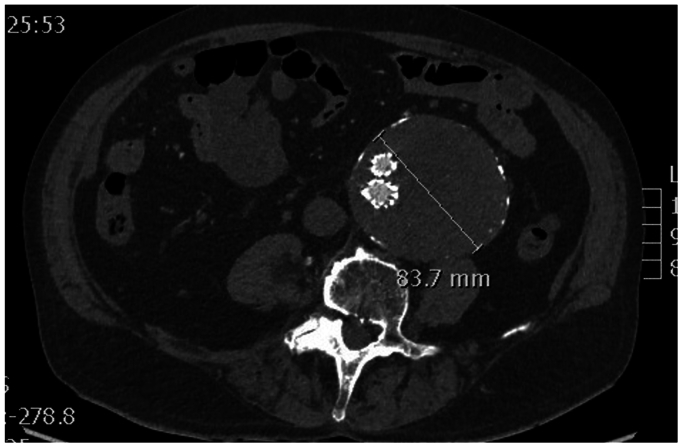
Computed tomography (CT) control scan after 1 year from inferior mesenteric artery coiling.
